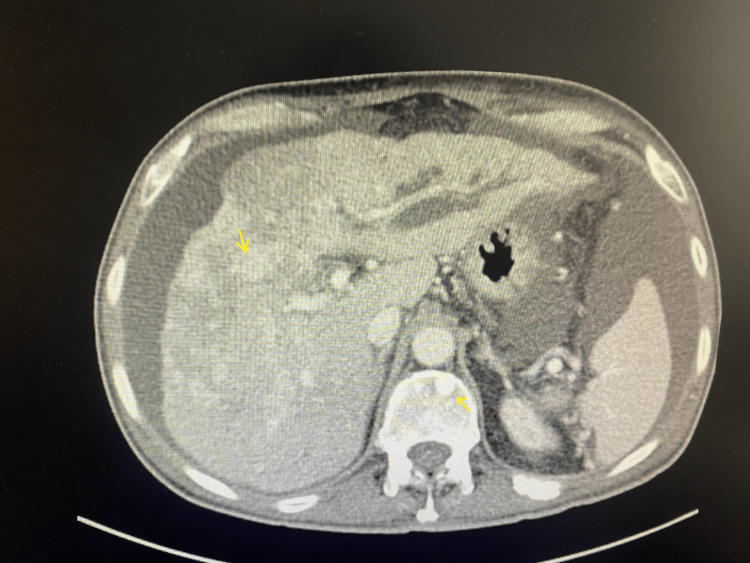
Liver and vertebral metastases in a 70-year-old male patient with medullary cancer (computed tomography).
